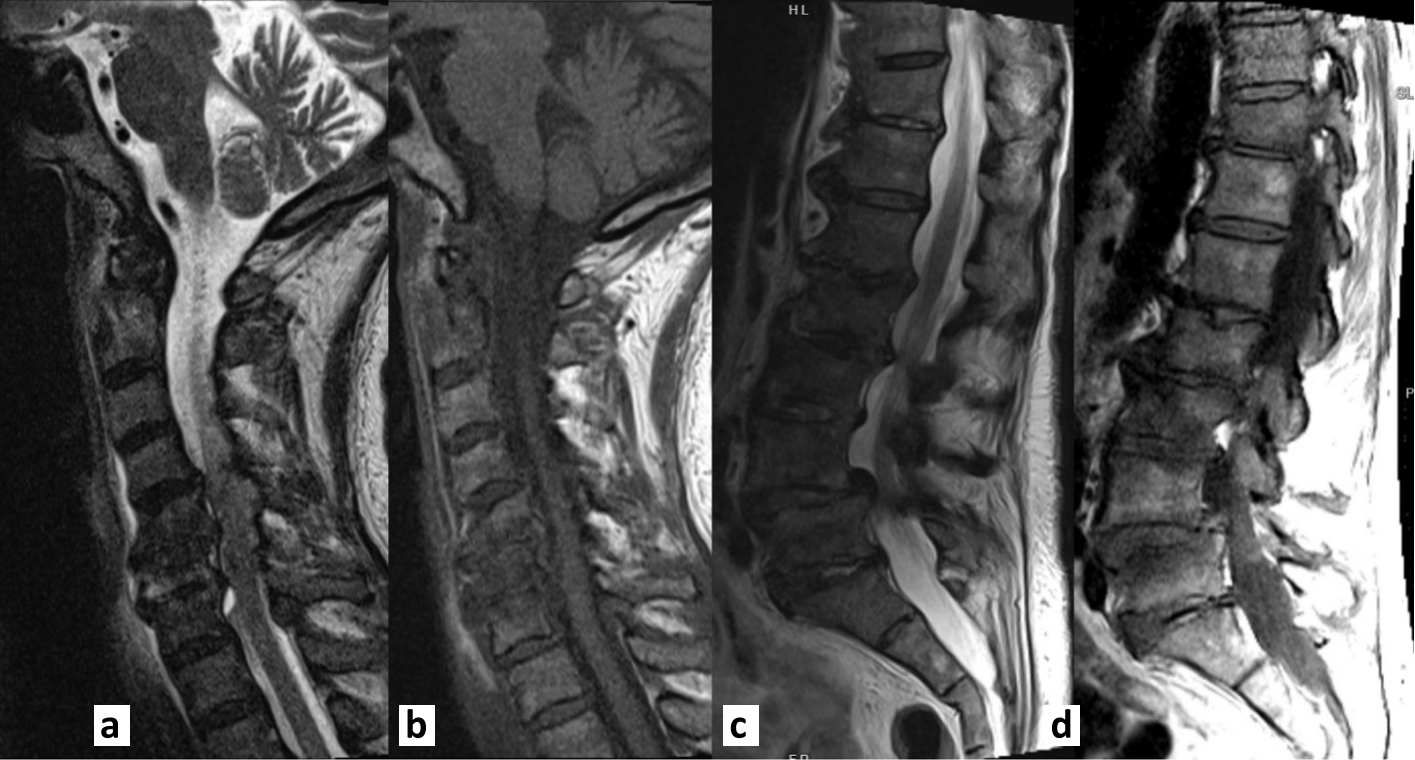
Magnetic resonance imaging of the cervical and lumbar spines. a-b T1 and T2 images of cervical spine showing endplate edema at C6-C7 level. Chronic degenerative changes without cord compression. c-d T1 and T2 images of cervical spine showing type I Modic change and enhancement along the endplates involving L2-L3 through L4–5, slightly increased at the L4-L5. Fluid signal intensity within the L3-L4 and L5-S1 disc levels with lesser involvement of L4-L5, L1-L2, and L2–3
